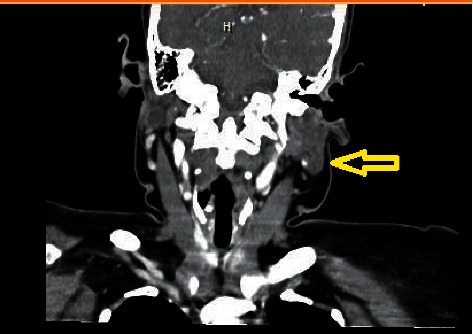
Neck soft tissue computed tomography revealing the left thyroid nodule.
